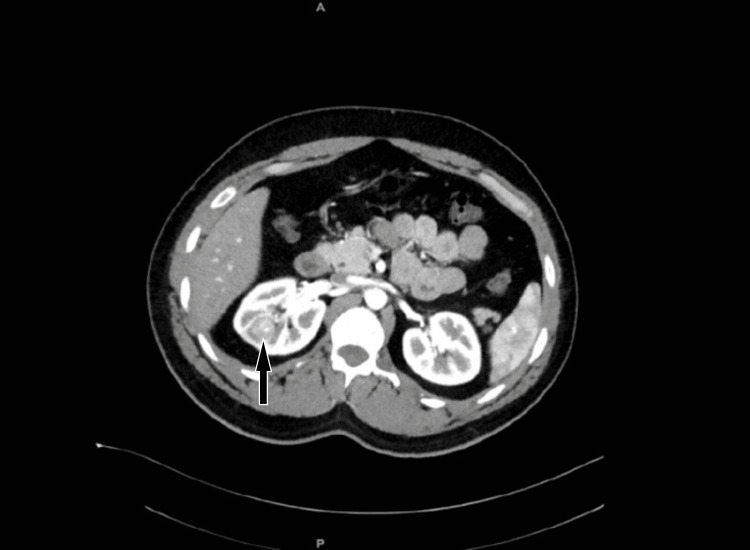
Contrast CT scan of the abdomen and pelvis, axial slice There is a right renal mid to upper pole enhancing renal mass measuring 1.8 x 2 x 2.2 cm.
